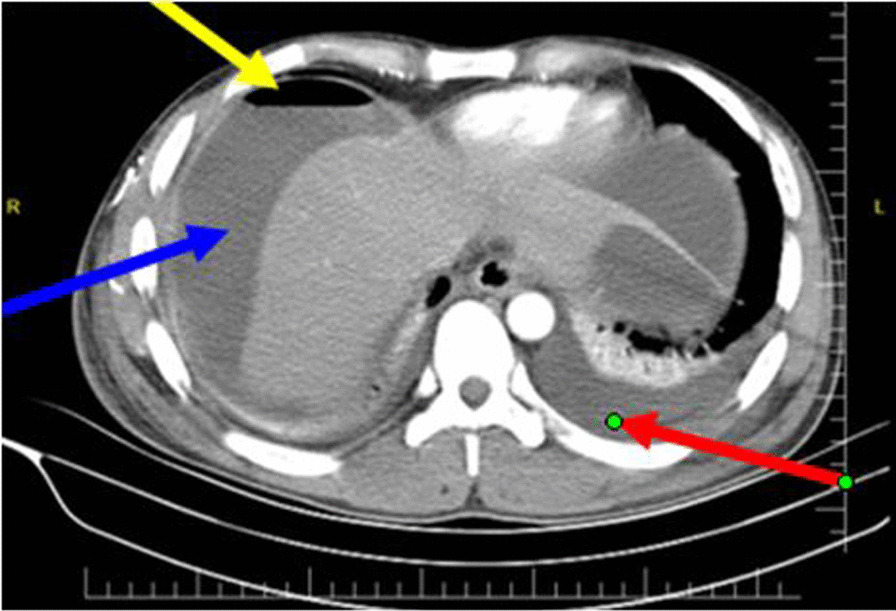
An axial abdominal CT showing free sub-diaphragmatic with air–fluid levels under right hemidiaphragm (yellow arrow), extensive free intraperitoneal fluid (blue arrow), and left pleural effusion (red arrow)
